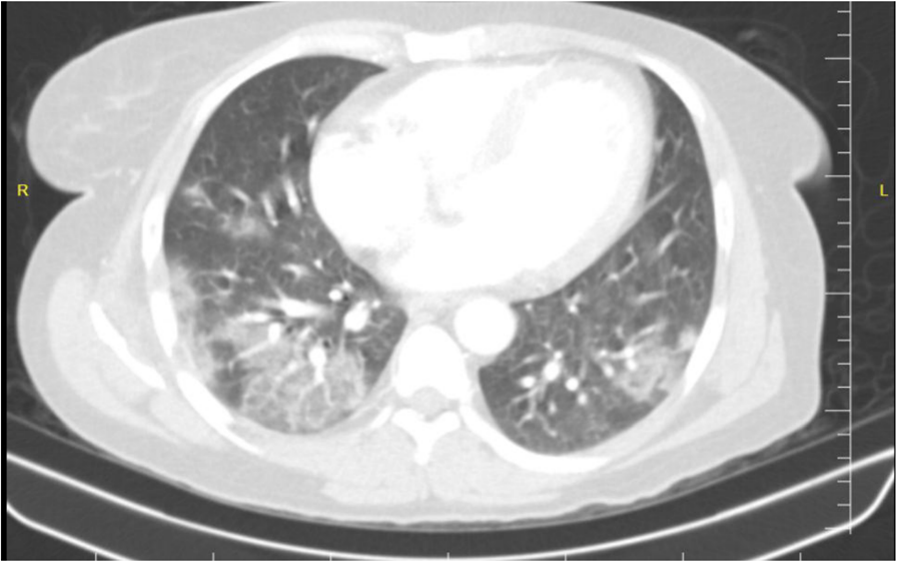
An axial chest CT from a 47-year-old woman with COVİD-19 pneumonia. Non-contrast-enhanced chest CT image showing multiple peripheral ground-glass opacities distributed in the bilateral multiple lobular and subsegmental areas
